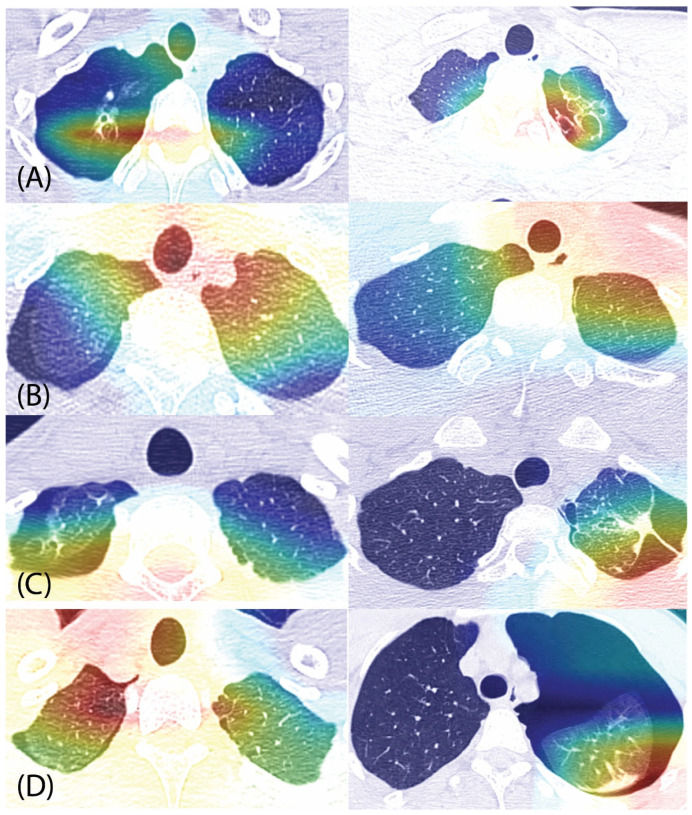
Gradient-weighted Class Activation Mapping (Grad-CAM) visualizations highlighting chest CT regions associated with recurrence of primary spontaneous pneumothorax (SP). Apical lung regions were frequently activated in cases with recurrence, particularly in the presence of definitive blebs or bullae. Notably, in recurrent cases without identifiable blebs or bullae, apical activation was still observed, suggesting a potential subclinical contribution. Panels: (A) recurrence with blebs or bullae; (B) recurrence without blebs or bullae; (C) non-recurrence with blebs or bullae; (D) non-recurrence without blebs or bullae.
